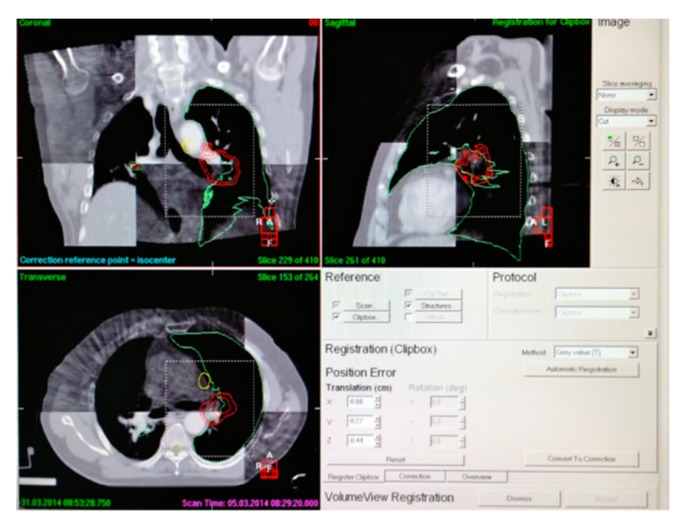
Cone beam computed tomography (CBCT) image calibration and positioning error calculation.
